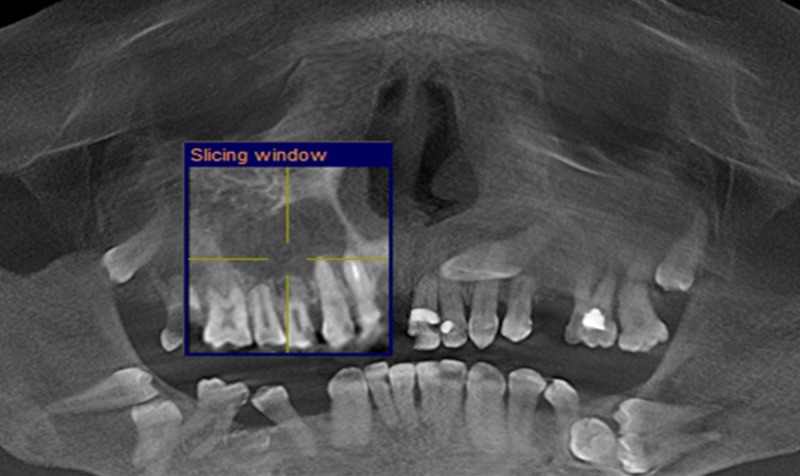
Unicystic radiolucency extending from the periapical area of 13 to the periapex of the 16 and the ‎complete absence of the maxillary sinus on the left side
